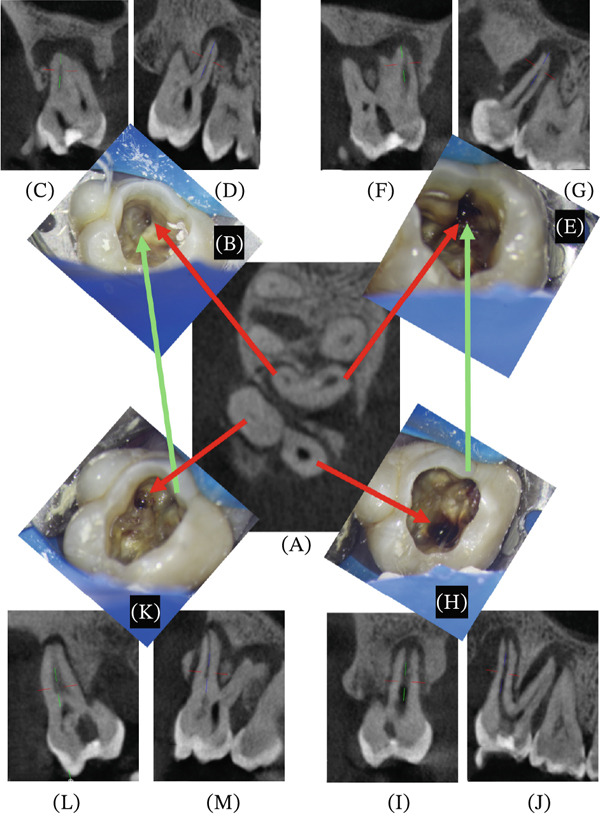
Tooth #2 with parastyle: mapping of internal anatomy of the pulp chamber, in association with the location of the root canal orifices under magnification, an axial CBCT section and centred sagittal and coronal sections of the root canals. (A) Axial CBCT section. (B) MB root canal orifice. (C) CBCT coronal section of the MB root. (D) CBCT sagittal section of the MB root. (E) MP root canal orifice. (F) CBCT coronal section of the MP root. (G) CBCT sagittal section of the MP root. (H) P root canal orifice. (I) CBCT coronal section of the P root. (J) CBCT sagittal section of the P root. (K) Parastyle root canal orifice. (L) CBCT coronal section of the parastyle root. (M) CBCT sagittal section of the parastyle root.
